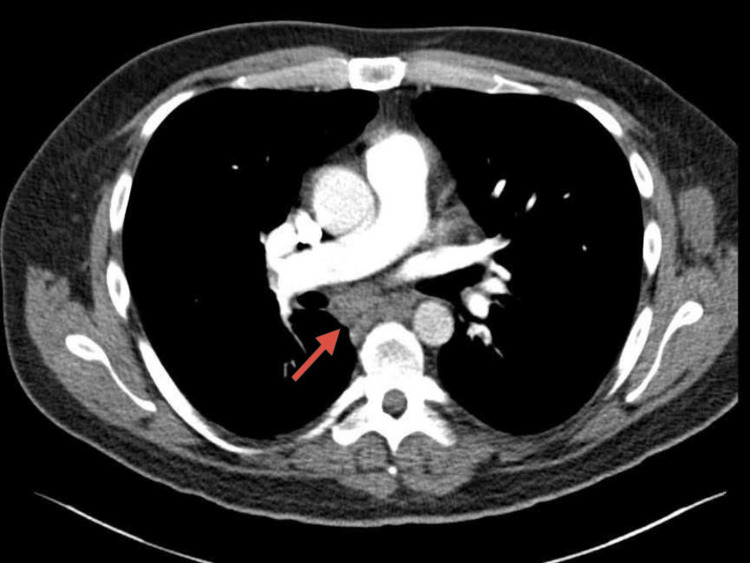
Axial chest CT scan showing pathologically enlarged mediastinal lymph nodes (red arrow), with the largest measuring approximately 2 cm in the left paratracheal region.
